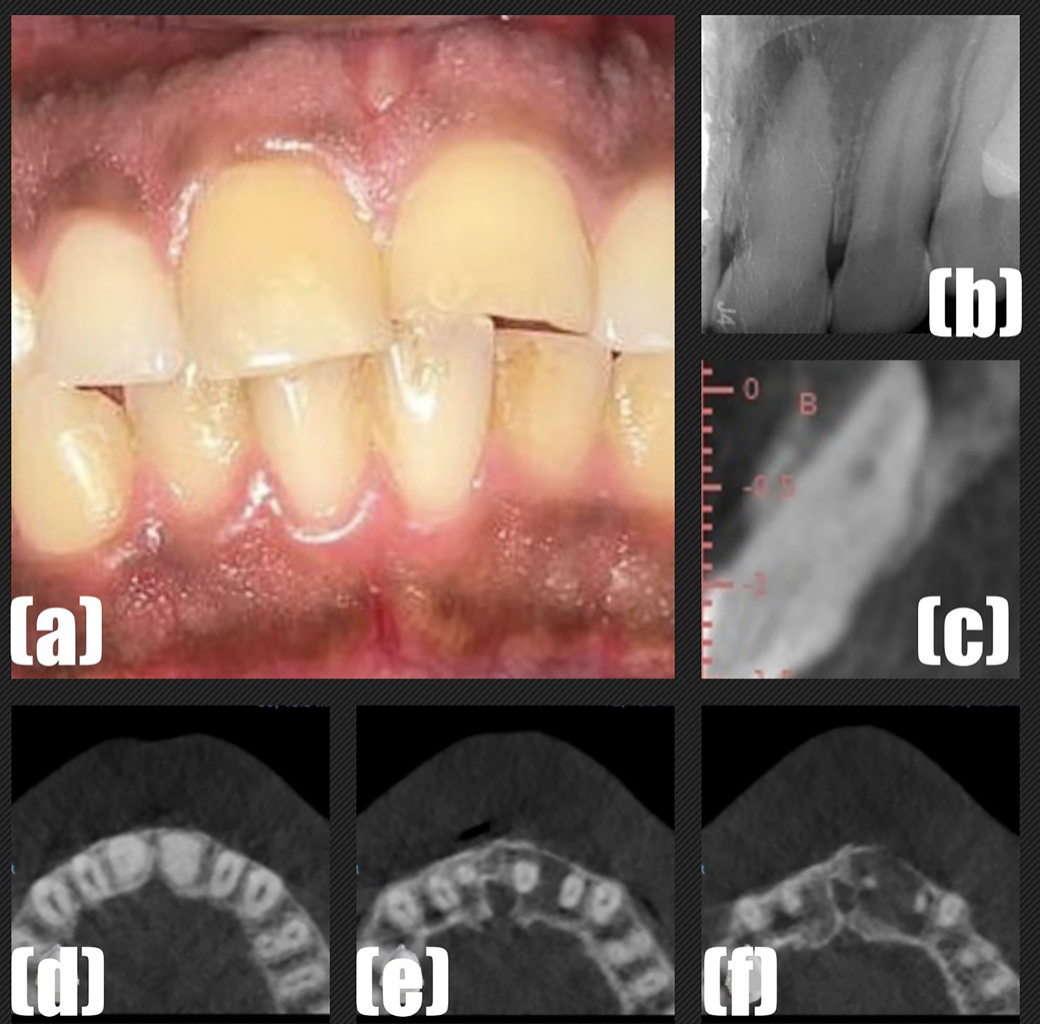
(a) Clinical aspect of teeth. (b) The periapical radiography which revealed PCC of #21 tooth and periapical radiolucency in relation to teeth #21 and #22. (c-e) CBCT showing PCC of teeth #11 and #21.
